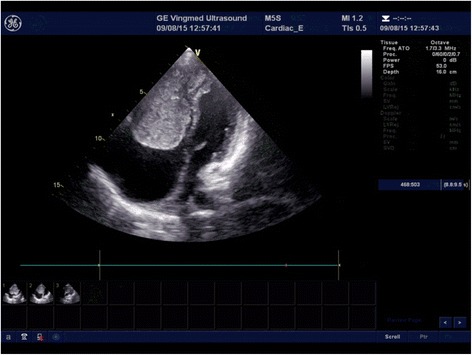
Transthoracic echocardiogram showing *right* ventricular mass
